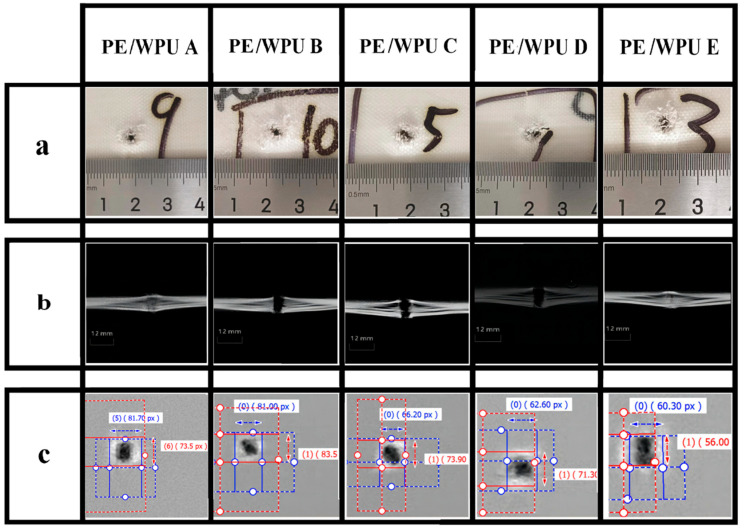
Characterization of the target plate penetration area: (a) picture of the impact face damage; (b) CT scan of interlayer destruction; (c) digital radiography of impact face.
